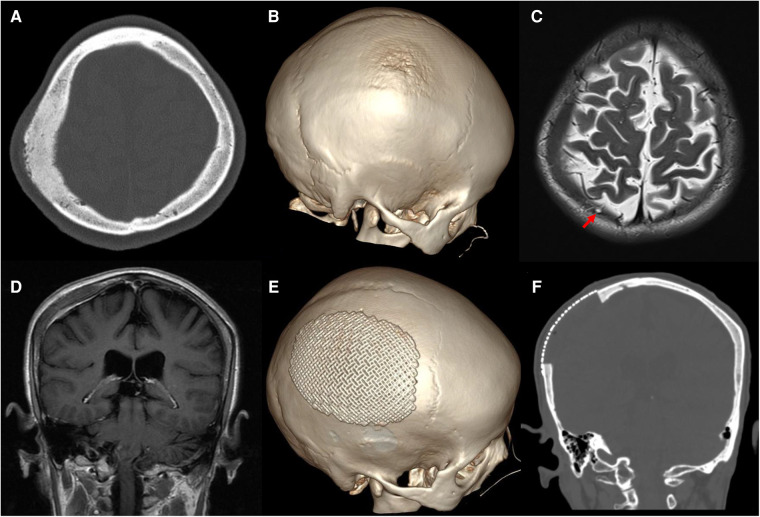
Preoperative images: (A) axial thin-cut skull CT shows an osteogenic lesion in the right parietal bone. (B) 3D-CT shows crater-like changes in the outer layer of the skull. Axial T2 imaging revealed a hypointense intraosseous lesion and arachnoid granules protruding into the diseased bone [(C), red arrow]. (D) Coronal gadolinium-enhanced MR imaging exhibits mild to moderate homogeneous enhancement with an intense dural reaction. Postoperative CT shows total resection of the tumorized calvaria lesion with good coverage by a titanium mesh (E,F).
